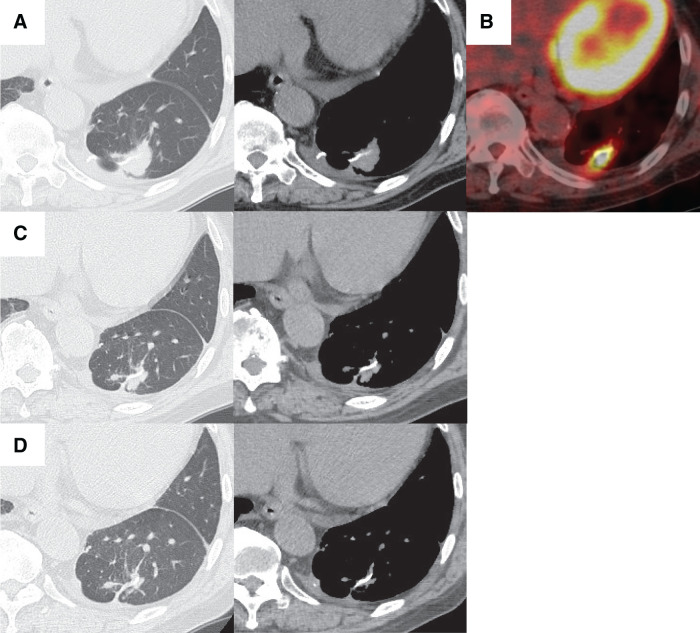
Postoperative CT images of Case 2. A nodule adjacent to the staple line was found on the postoperative CT scan, which was obtained 8 months after surgery (A). The SUVmax of FDG uptake on PET/CT was 10.3 (B). The nodule shrank in size on the CT scan, which was performed 1 month after administration of tranilast (C), and the nodule had disappeared by the 4th month after tranilast administration (D). FDG, fluorodeoxyglucose; PET, positron emission tomography; SUVmax, maximum standardized uptake value
